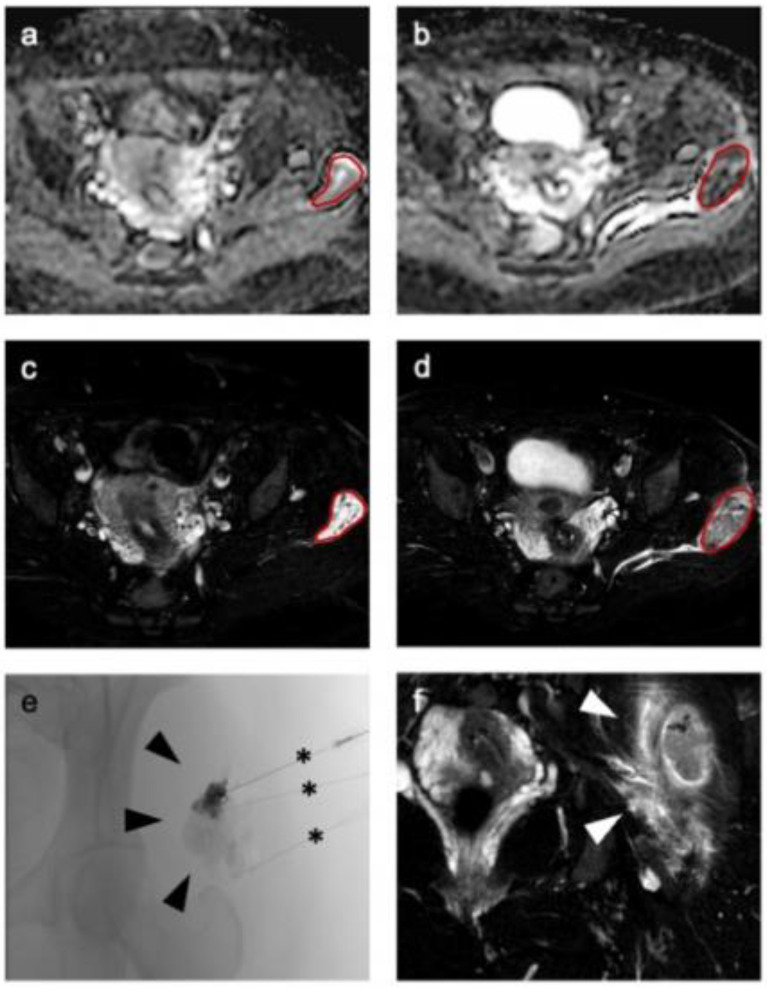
Representative imaging of a venous malformation in the gluteal area of a 34-year-old woman before therapy (a) shows a well marginated lesion on apparent diffusion coefficient (ADC) (a) and T2-weighted (c) imaging, which is markedly hypo-intense after therapy in ADC (b) and T2-weighted imaging (d,f) with a surrounding hyperintense edema (arrowheads in (f)). For segmentation a volume of interest (red line) was drawn on T2-weighted images (c,d) and transferred to ADC (a,b). (e) Representative fluoroscopic imaging during percutaneous sclerotherapy followed by administration of the sclerosing agent via percutaneous needles in three locations (black asterisks).
